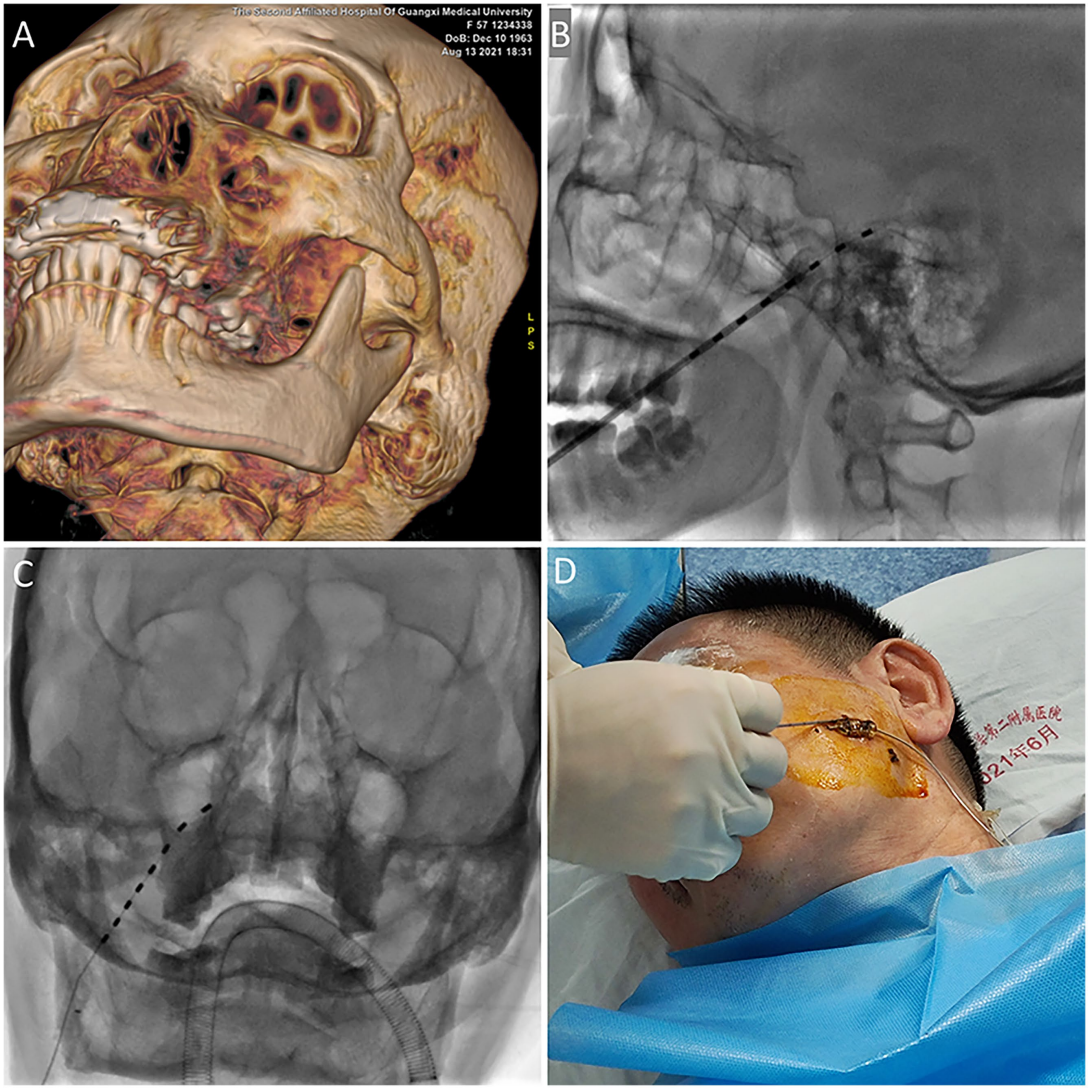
(A) The foramen ovale was identified through three-dimensional reconstruction. (B) The electrode was advanced until the tip of the most distal contact reached the clivus. (C) An anteroposterior radiographic image of the percutaneous TGS with lead placement is presented. (D) The electrode was anchored using a soft silastic anchor. TGS: trigeminal ganglion stimulation.
